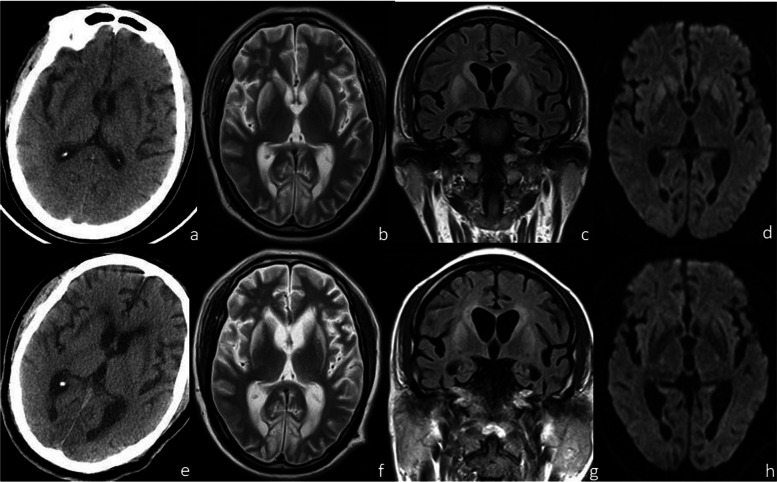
A 49-year-old male who had unconsciousness and of limb tics after H_2_S inhalation in a fish hold was admitted to our hospital after 51 days of treatment in another hospital and was still in a coma and had hypermyotonia in the four limbs on admission. CT of the brain was performed 53 days after the poisoning and revealed symmetrical stripes and patchy hypodensities in the bilateral basal ganglia (a); MRI on the same day revealed symmetrical hypointensities on T1WI and hyperintensities on T2WI around the lateral ventricles and in the bilateral basal ganglia (putamen and head of the caudate nucleus) (b), and the lesions were slightly hyperintense on T2WI-FLAIR (c) and DWI (d); CT of the brain 93 days after the poisoning was comparable to the previous CT (e), and MRI on the same day revealed that the ranges of lesions on T2WI (f) and T2WI-FLAIR (g) were also comparable to those on the previous MRI, but reduced hyperintensities were revealed on DWI (h); MRI of the brain performed 6 months after poisoning revealed that there were still no significant changes in the lesions. The patient had a poor prognosis and was in a persistent coma
